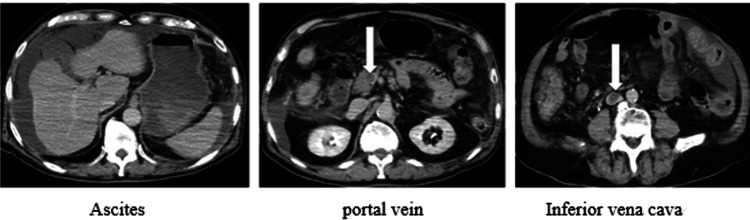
The abdominal CT images on day 1 of admission. Thrombi are observed at the tips of the arrows. CT, computed tomography
